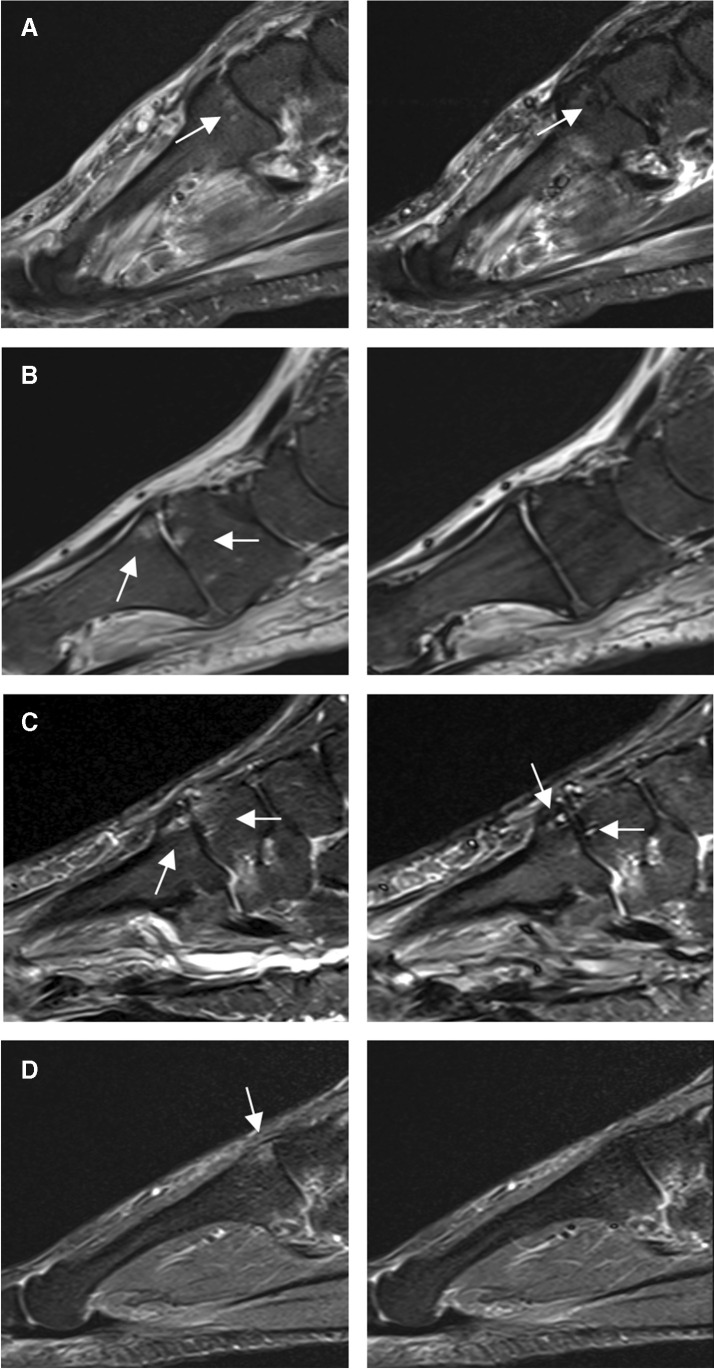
Obscured bone marrow edema-like signal on postcontrast STIR images. Precontrast STIR images of four different patients (A to D) with bone marrow edema-like signal at the Lisfranc joint (left) show signal suppression on postcontrast STIR images (right). A 1.5-T MRI of an 84-year-old male patient shows bone marrow edema-like signal at the base of the second metatarsal bone on precontrast STIR (left, white arrow). The signal intensity of bone marrow edema-like signal is reduced even below the adjacent normal appearing bone on the corresponding postcontrast STIR image (right, white arrow). B 1.5-T MRI of a 94-year-old male patient with bone marrow edema-like signal on both sides of the Lisfranc joint of the first ray (left, white arrow) on precontrast STIR with complete suppression of the signal on postcontrast STIR (right). C 3-T MRI of a 69-year-old female patient shows bone marrow edema-like signal with cystic changes at the Lisfranc joint of the second ray on precontrast STIR (left, white arrow) with nearly complete signal suppression on postcontrast STIR (right). Note the persisting high signal of the cystic changes on the postcontrast STIR image (white arrow). D 1.5-T MRI of a 37-year-old female patient with bone marrow edema-like signal at the base of the second metatarsal bone on precontrast STIR (left, white arrow) with complete signal suppression on the postcontrast STIR image (right)
